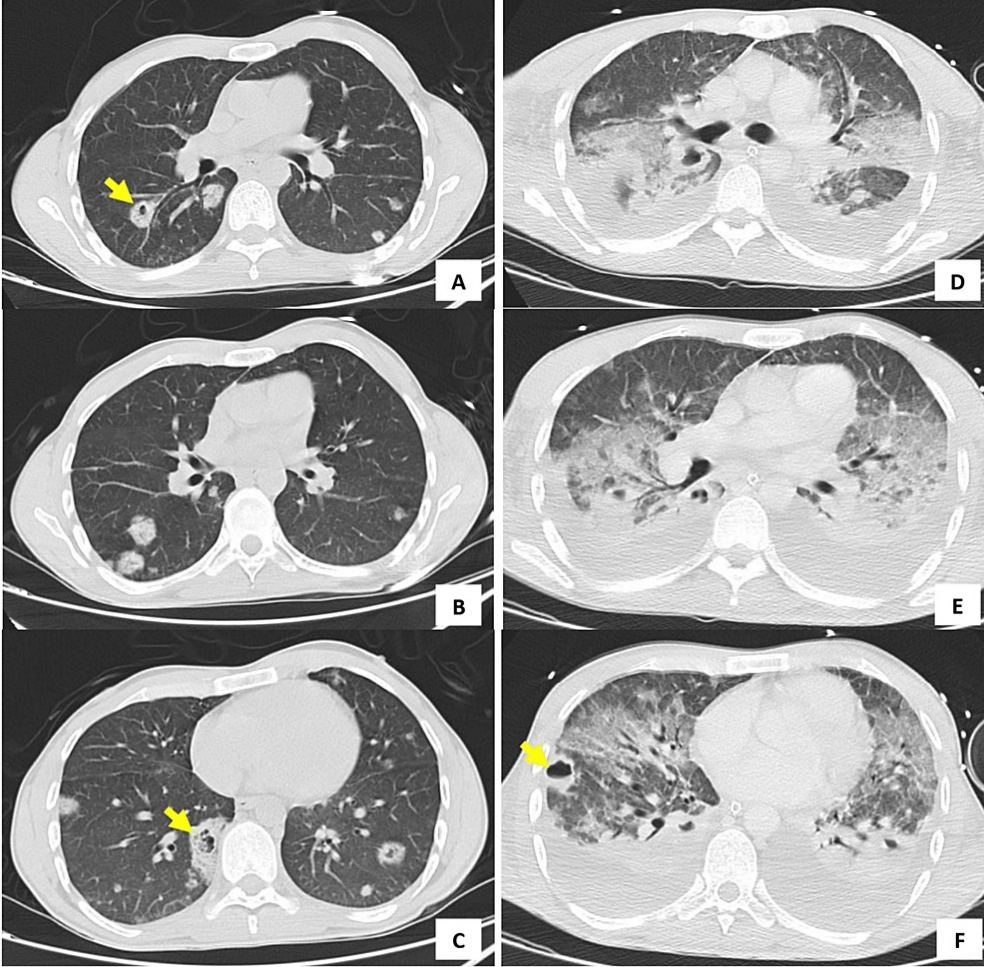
A CT thoracic scan shows septic pulmonary emboli at hospital admission (panel A, B, C) and at intensive care admission one week later (panel D, E, F). The arrows show pulmonary cavities.
